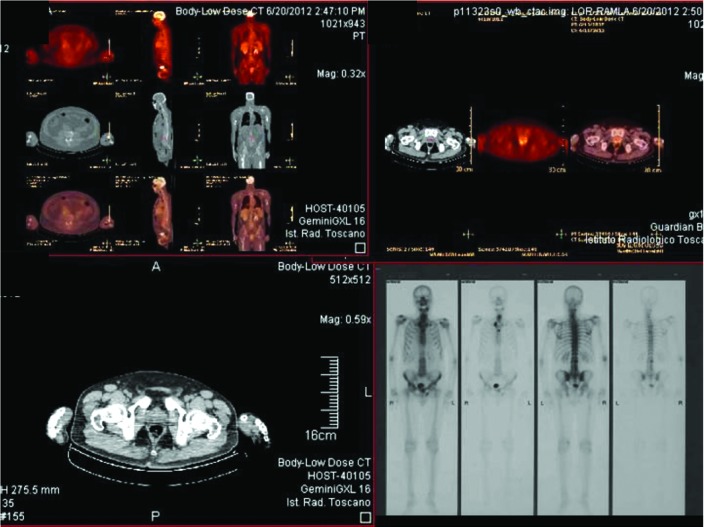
(^18^F-FDG-3′5MBq) fluorodeoxyglucose-positron emission tomography (FDG-PET) of case 4. FGD-PET prostate positive signaling was the only sign of prostate cancer that was identified during medical investigations due to other causes.
